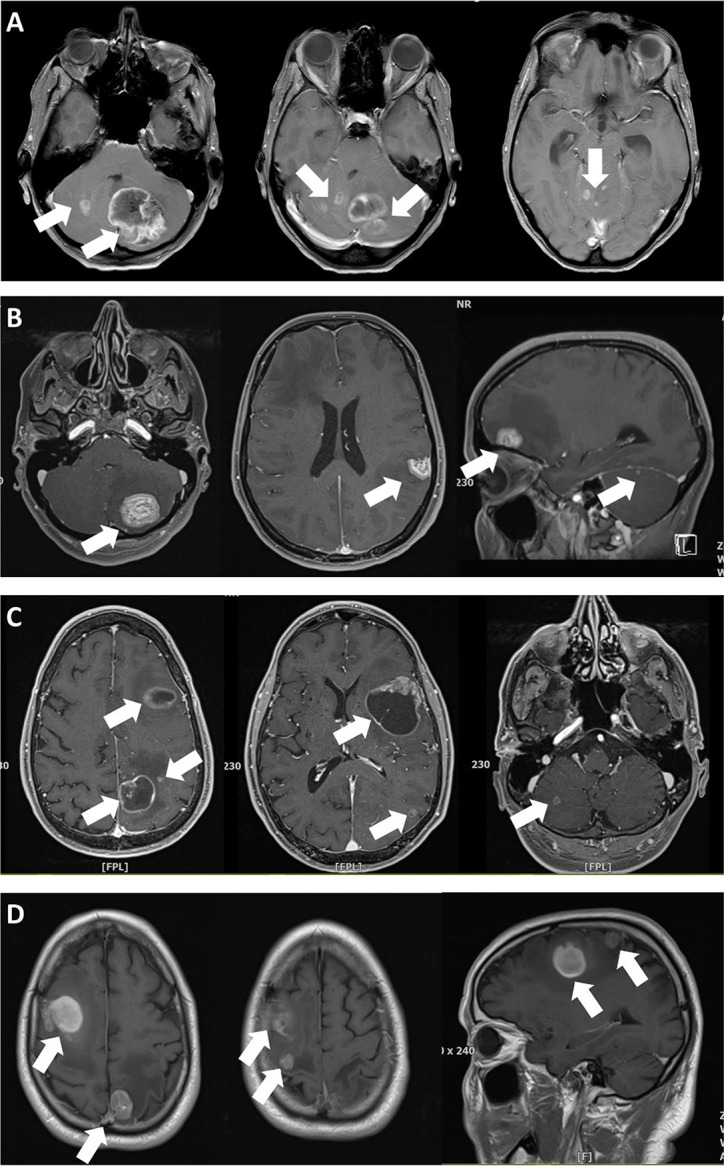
Examples of surgical indications (T1 weighted & contrast-enhanced MR images) a 51-year-old female patient with multiple brain metastases in the posterior fossa (arrows) and known esophageal cancer. Surgery was performed primarily in order to avoid occlusive hydrocephalus, i.e. only the large left mass consisting of several coalescing metastases was removed. The patient survived for 34 months following the operation. b 35-year-old female with breast cancer (ER+, PR+, HER2+), hepatic metastases diagnosed 41 months earlier and four brain metastases (arrows). She presented with headaches and a visual field cut. The treating oncologist requested tissue for molecular analyses (“molecularly informed treatment”). We felt that this case might benefit from aggressive local treatment of all CNS cancer manifestations. Hence, the patient had two surgeries for removal (1) of the left cerebellar and temporo-parietal metastases and (2) the right fronto-basal tumor. All tumor were HER+, but ER-/PR-. The small right cerebellar metastasis was treated with radiosurgery. We favored surgery for the frontal and the temporo-parietal lesions in order to save radiosurgery as an option for tumor recurrence. Indeed, despite adjuvant radiotherapy further radiosurgeries and even one additional surgery for a left cerebellar recurrence were required later on for CNS disease control. The patient was alive and well at her last follow-up 30 months after the initial operations. C 58-year-old male patient presenting with multiple brain metastases (arrows; additional small tumors in the left temporal and both occipital lobes), mild aphasia and no known primary tumor. Initial staging investigations suggested lung cancer as the primary tumor. However, a bronchoscopy failed to establish a tissue diagnosis. Awake surgery was performed for removal of the left frontal lesion in order to relieve the mass effect and its consecutive neurological symptoms (aphasia) and to obtain a tissue diagnosis. The patient improved and was neurologically intact after the surgery. Pathological evaluation of the CNS metastasis revealed carcinoma with neuroendocrine differentiation well in line with the presumptive diagnosis of primary lung cancer. d 66-year-old male patient diagnosed with malignant melanoma 10 years earlier and presenting with moderate paresis of the right hand > arm. The tumors (arrows) were removed through two separate craniotomies with the help of intraoperative MEP neuromonitoring. Surgery was favored over primary radiotherapy/-surgery because of the presumed resistance of malignant melanoma CNS metastases to radiotherapy and in an attempt to preserve the patient’s neurological status though removal of the lesion in the right precentral gyrus. The patient’s neurodeficit indeed improved slightly after surgery
